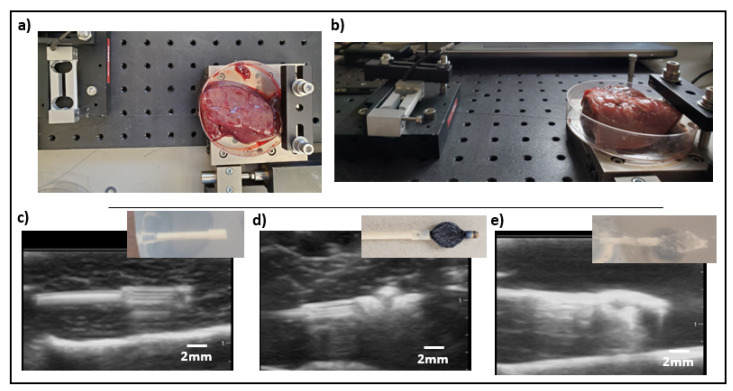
Representative force testing set-up in liver (a) and kidney (b) samples. Non-anchored (c), nitinol-anchored (d), and hydrogel-anchored (e) microdevices are well seen by ultrasound in all tissues at the implanted depths. Insets show the actual appearance of each device corresponding to its ultrasound image. Ultrasound was used to monitor for microdevice dislodgement in all solid tissues.
